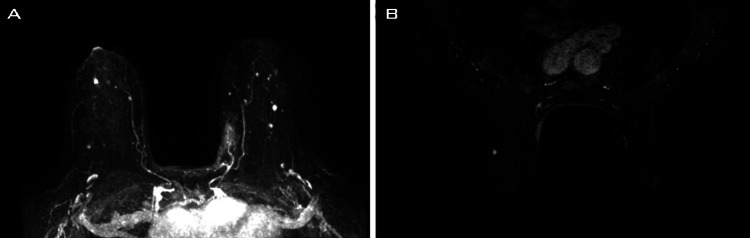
Breast contrast-enhanced MRI (a) MIP reconstruction shows bilateral multiple diffusely-distributed oval-shaped nodules with well-defined borders and marked homogeneous enhancement; (b) Subtraction imaging shows the biggest nodule at the upper inner quadrant, in which biopsy was performed. MIP: maximum intensity projection
